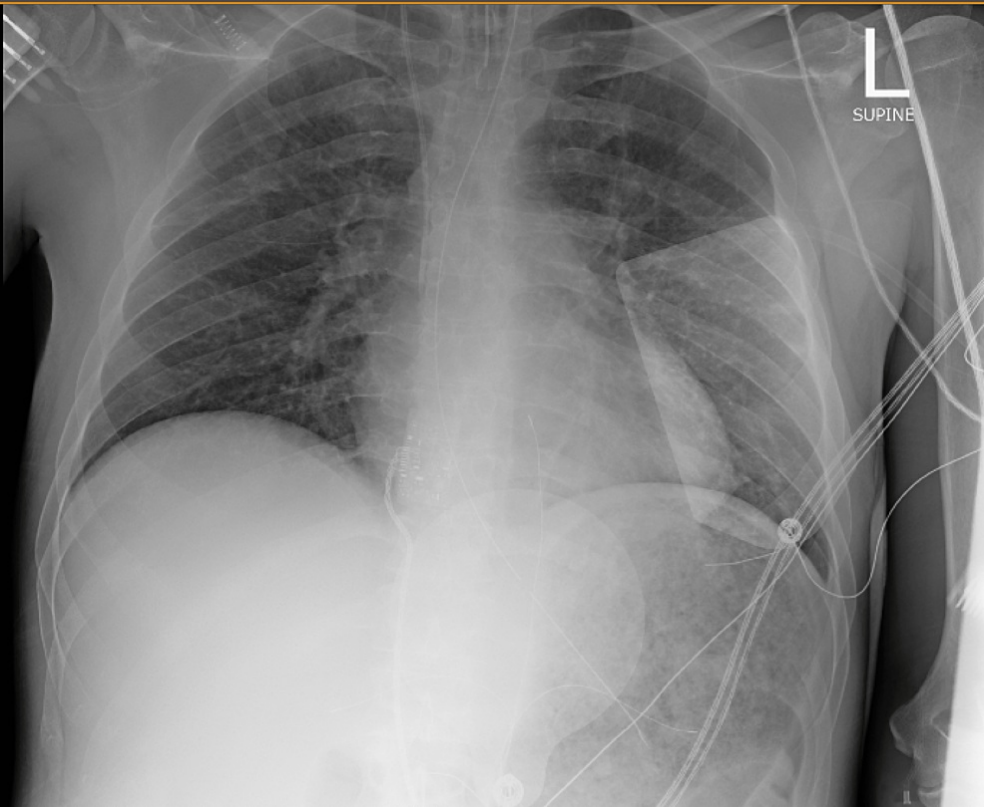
Chest radiograph in the anterior-posterior (AP) view showing a subtle opacity in the right upper lobe
